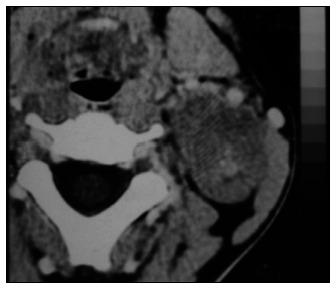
Cervical computed tomography scan showing schwannoma in sympathetic chain.
